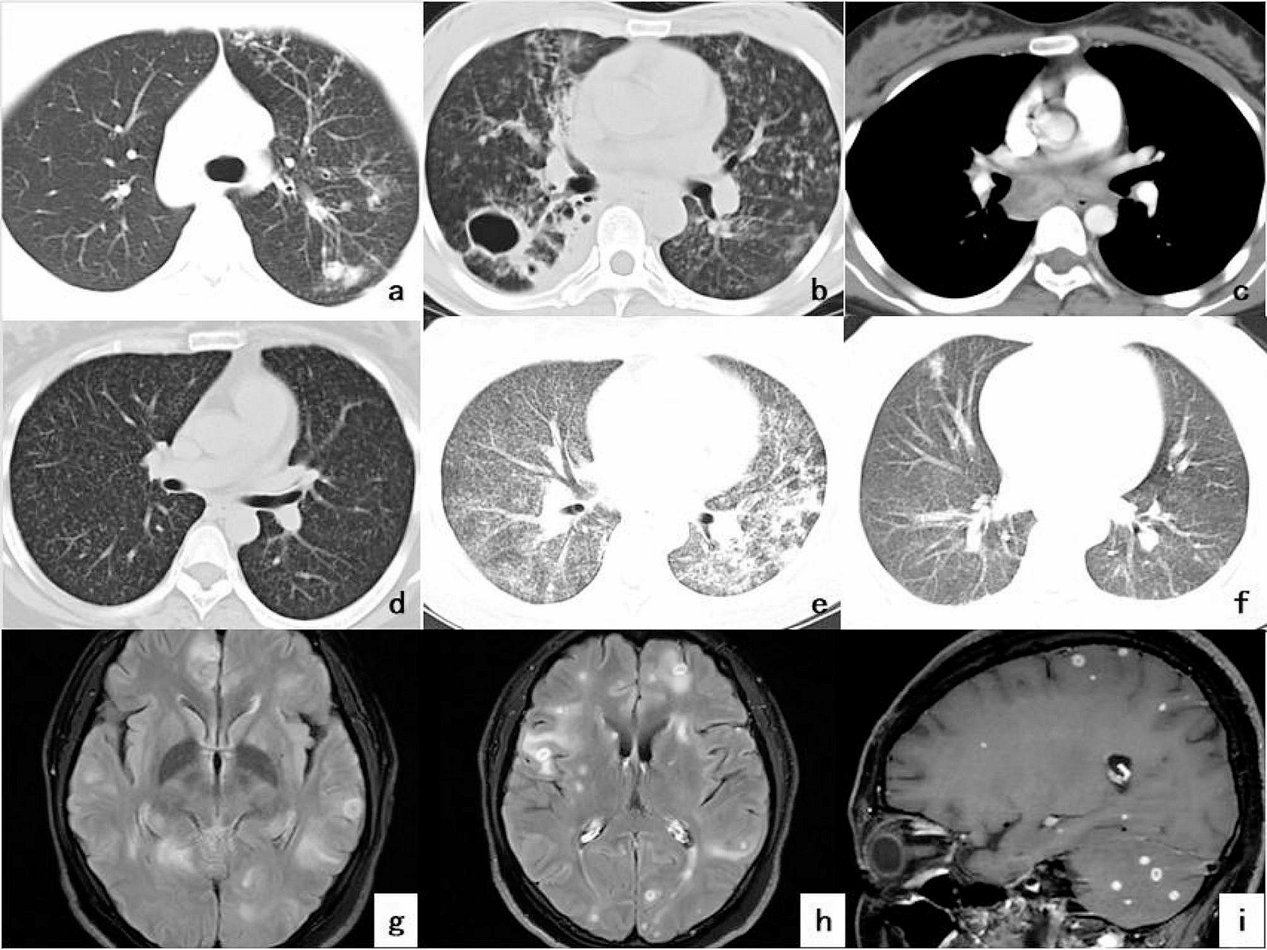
Imaging findings of pregnancy complicated with pulmonary tuberculosis and intracranial tuberculosis. a. shows the nodular and patchy shadows of the left lung, with local Tree-in-Bud sign; b. The cavity and multiple patchy consolidation in the right lung; c. Enlargement of intrathoracic lymph nodes, and the ring enhancement of enhancement scan; d-f. Three different patients of acute hematogenous disseminated tuberculosis, presenting a “three uniform” millet nodule shadow, partial patchy fusion; g-i. MRI findings in the same patient; g. the T2Flair transverse position. Multiple intracranial nodules with slightly higher T2 signal and partial peripheral brain parenchyma edema; h. For transverse images of T2Flair enhancement. Focal nodular and annular enhancement; i. Sagittal images T1WI enhancement. Focal nodular and annular enhancement
